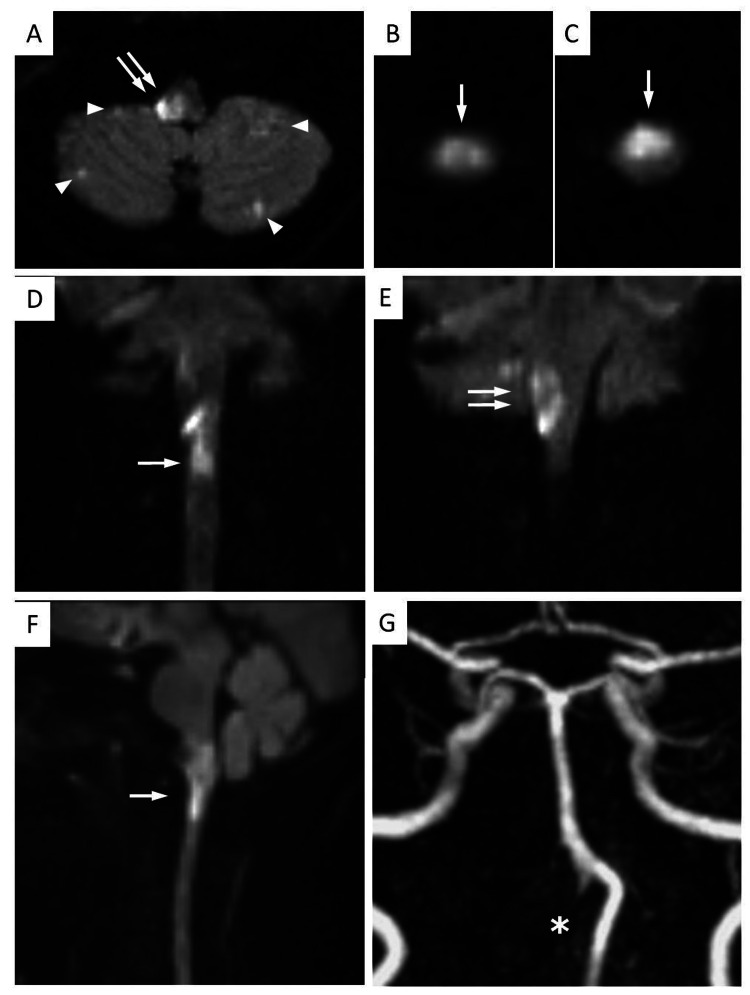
Postoperative MRI images. (A) Intracranial MRI (DWI) obtained three days after intervention shows right lateral medullary infarction (double arrow) and ischemic spots in bilateral cerebellum (arrowheads). (B and C) Cervical MRI (DWI, axial section) shows high intensities in the medial and anterior spinal cord (arrow). (D-F) Cervical MRI (DWI) (D, E: coronal, and F: sagittal view) shows right lateral medullary infarction (double arrow) and cervical spine infarction (arrow) at the level of C1/2. (G) MRA shows the right vertebral artery occlusion (asterisk).DWI, diffusion-weighted image; MRA, magnetic resonance angiography
